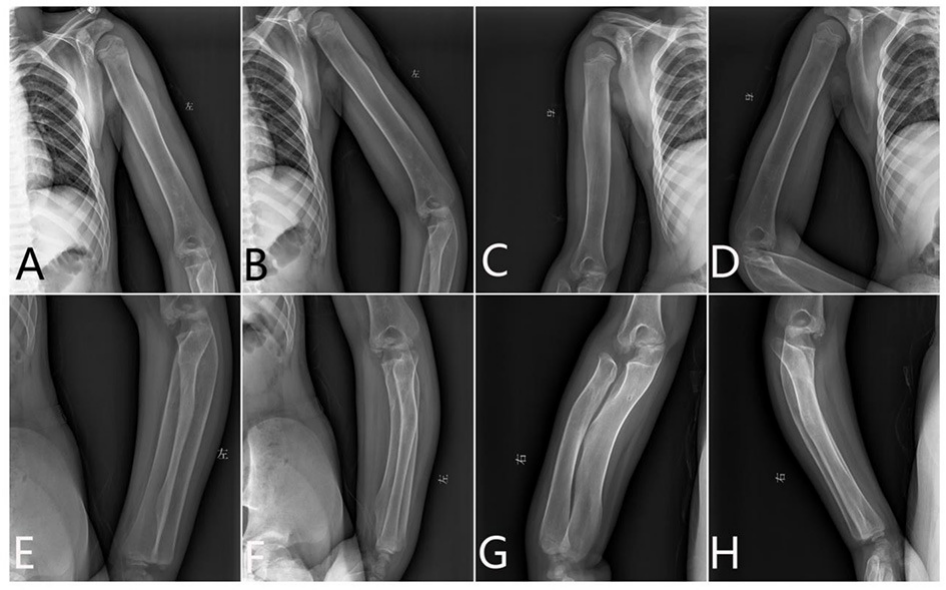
The x-ray radiography of the humerus, radius, and ulnas. (A,B) Left humerus, (C,D) right humerus, (E,F) left limb, and (G,H) right limb.
